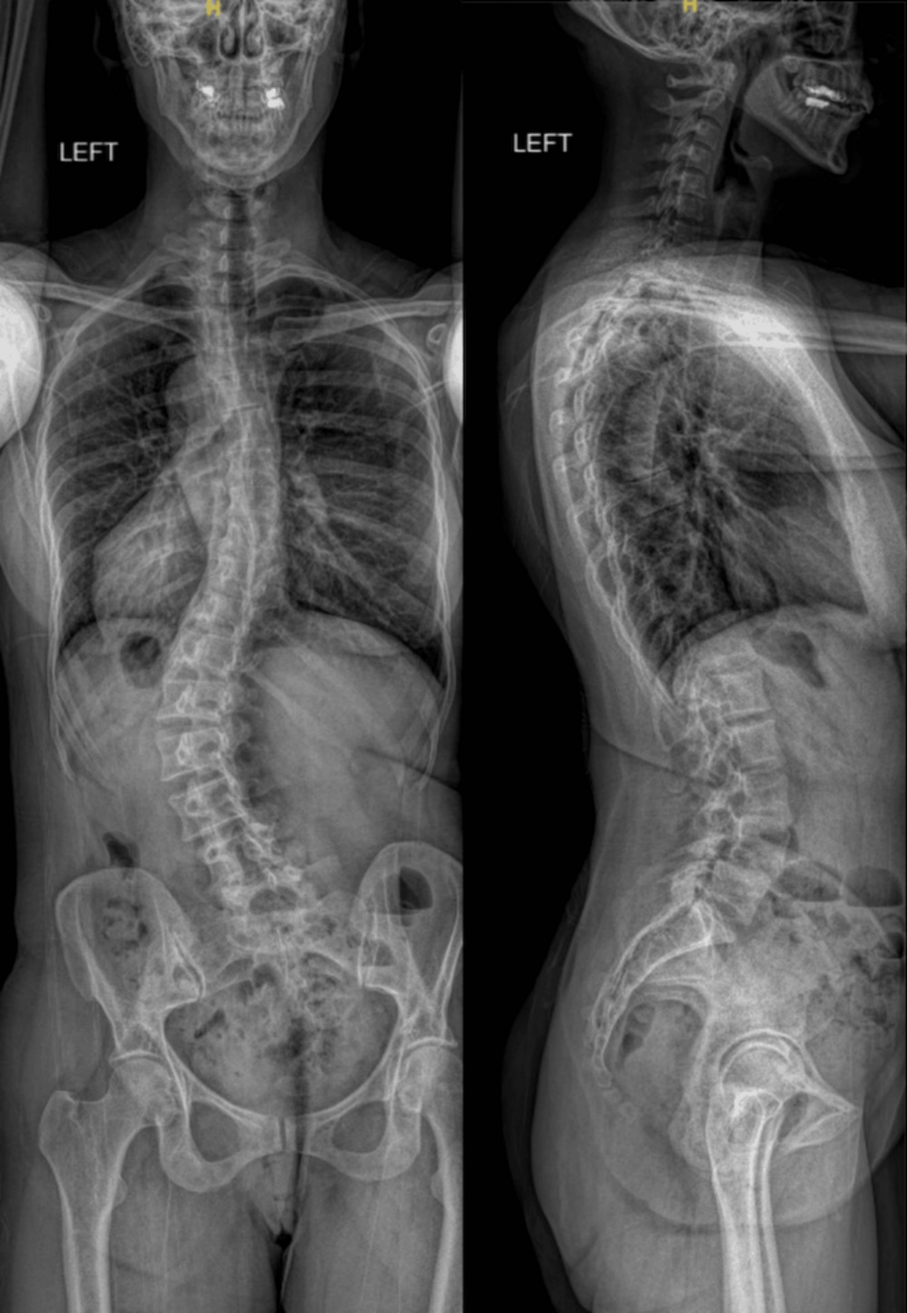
Preoperative anteroposterior (left) and lateral (right) long-cassette radiographs demonstrating levoscoliosis with a thoracolumbar curve apex at the L2 and associated coronal malalignment.
